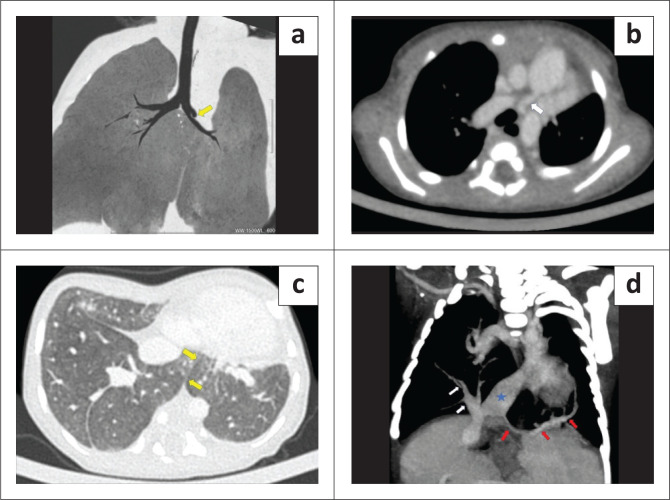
Pseudo-horseshoe lung in a 7-day-old male with left lung hypoplasia, total anomalous pulmonary venous return and multiple cardiovascular anomalies. (a) Coronal (minimum intensity projection [MinIP]) image of the contrast enhanced CT chest (lung window) demonstrates the rudimentary blind ending left upper lobe bronchus (arrow), absence of left upper lobe parenchyma and relatively narrow calibre of the left main bronchus in comparison with the right. (b) Axial maximum intensity projection (MIP) image shows the hypoplastic left pulmonary artery (arrow) arising from the main pulmonary artery. (c) Axial CT chest (lung window) demonstrates an isthmus of lung parenchyma joining the basal segments of both lungs posterior to the heart, with a thin intervening isthmic fissure (arrows). (d) Coronal oblique MIP image shows total anomalous pulmonary venous return with the right common anomalous pulmonary vein (white arrows) and the left single pulmonary vein (red arrows) both draining into the supra-hepatic inferior vena cava (star).
